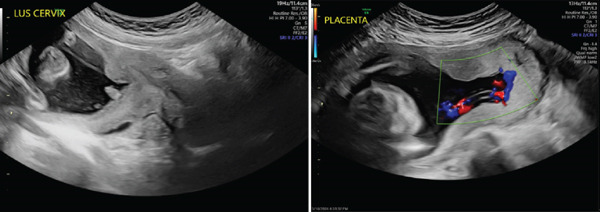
Ultrasound findings of the left lateral placenta with no placenta previa or PAS at 19 weeks′ gestation.
